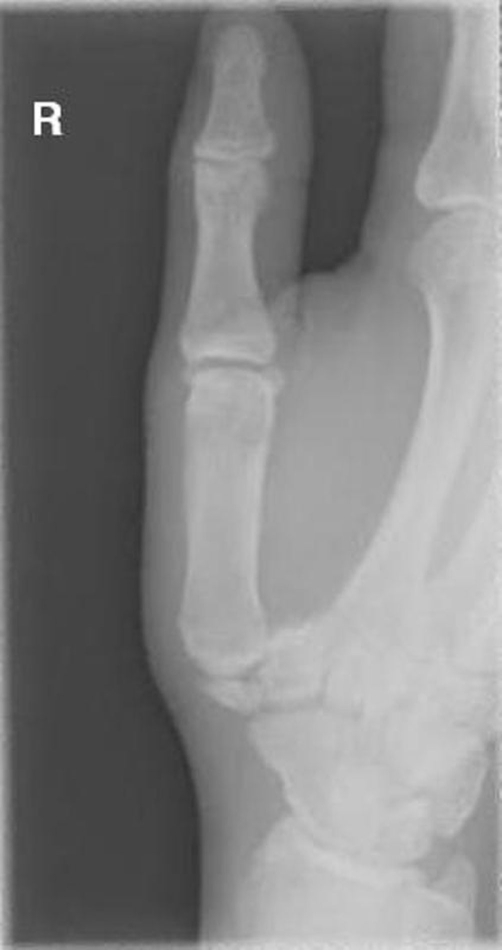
Plain radiograph of right hand post injury showing trapezium fracture and carpometacarpal dislocation.
